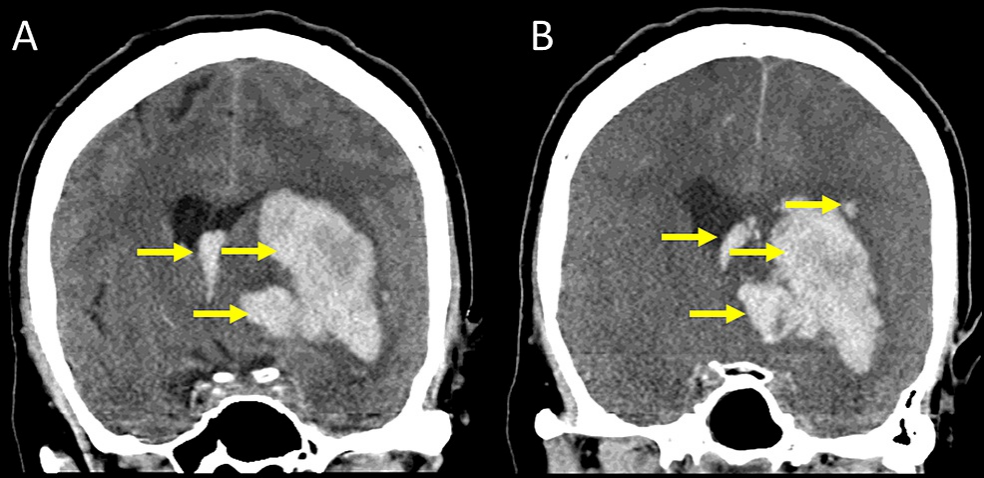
(A) Initial computed tomography (CT) scan taken in the coronal plane after the presentation to the emergency department. (B) A follow-up coronal CT scan of the head taken in the coronal plane five days later demonstrates minute differences, even after medical management.
